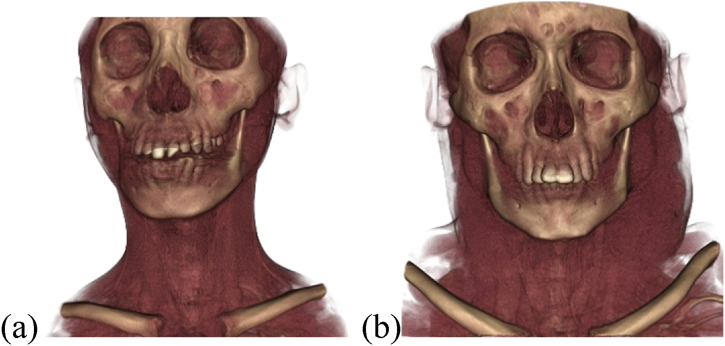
Three-dimensional CT scans. (a) subject 1; (b) subject 2.
